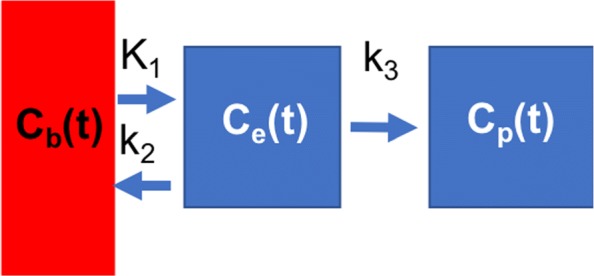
Irreversible two compartmental model describing the kinetics of ^18^F-FDG used to evaluate lung inflammation. In the absence of significant oedema, the concentration of ^18^F-FDG in a ROI in the lung can be described by three compartments [9]: a blood compartment *C*_*b*_(*t*), an extravascular pre-cursor pool *C*_*e*_(*t*) and phosphorylated (’trapped’) ^18^F-FDG compartment *C*_*p*_(*t*). The relationships between the concentration of tracer in a compartment is described by the rate constants (i.e. *K*_1_,*k*_2_,*k*_3_). *ROI* = *Region of Interest*
